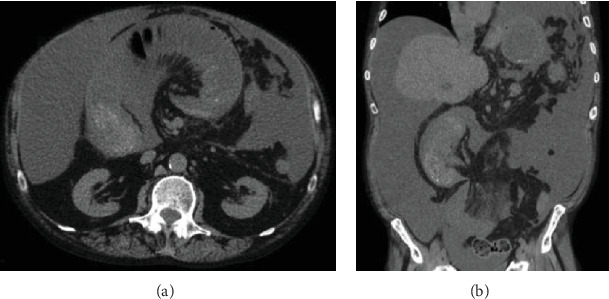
Abdominal computed tomography at the time of current hospital presentation in (a) transverse and (b) coronal planes demonstrating closed loop small-bowel obstruction in a known background of intestinal malrotation, cirrhosis, and ascites.
